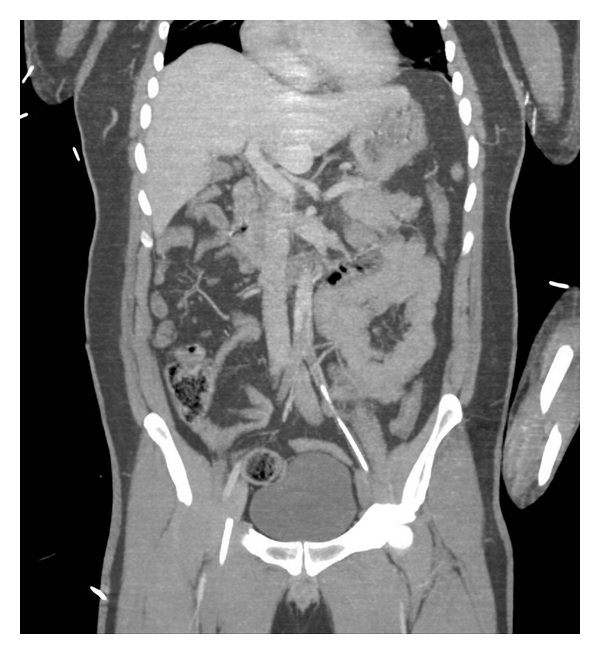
Abdominal CT showed multiple splenic and renal infarcts and a thrombus at the aortic bifurcation extending into the inferior mesenteric artery and into both common iliac arteries.
